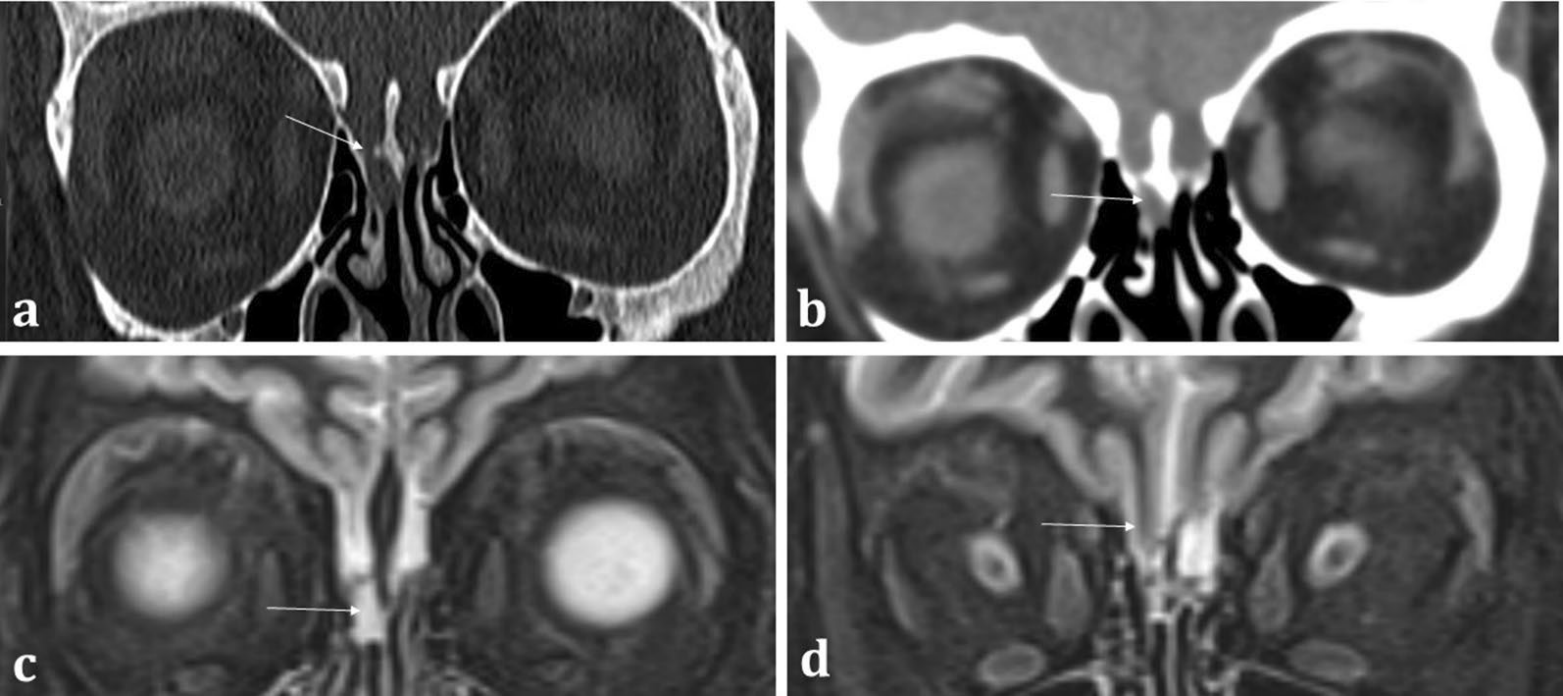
Acquired post-traumatic CSF fistulas in two different patients. Coronal bone (a) and soft tissue (b) CT images demonstrate a tiny bony defect of the right cribriform plate (arrow, a) and abnormal opacification of the right olfactory recess on the soft tissue window (arrow, b). The coronal T2WI MRI image of the same patient confirms herniation of fluid intensity CSF into the right olfactory recess consistent with a meningocele (arrow, c). For comparison, coronal T2WI MRI image of another patient (d) demonstrates herniation of both the gyrus rectus and CSF consistent with a meningoencephalocele (arrow, d)
